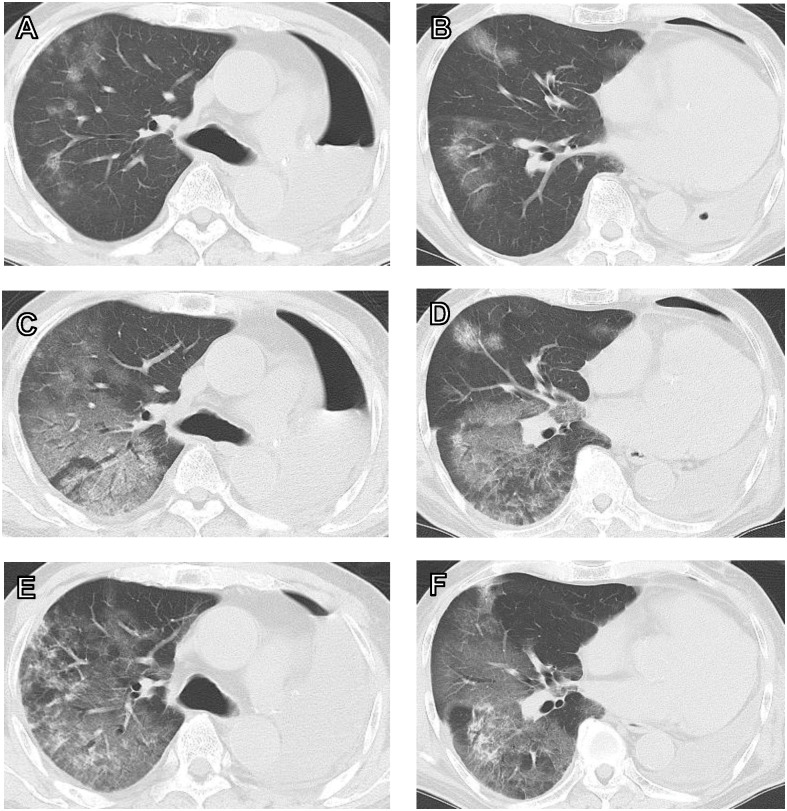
Chest CT findings during the clinical course. (A, B) Chest CT on day 40 at the unscheduled visit showing peripheral ground-glass opacities in the right lung, with patchy ground-glass opacities in the right upper and middle lobes and more extensive ground-glass opacities in the lower lobe. (C, D) Chest CT on day 42 showing rapid progression and more extensive ground-glass opacities in the right lung compared with day 40. (E, F) Chest CT on day 51 showing further progression of extensive ground-glass opacities in the right lung compared with day 42.
